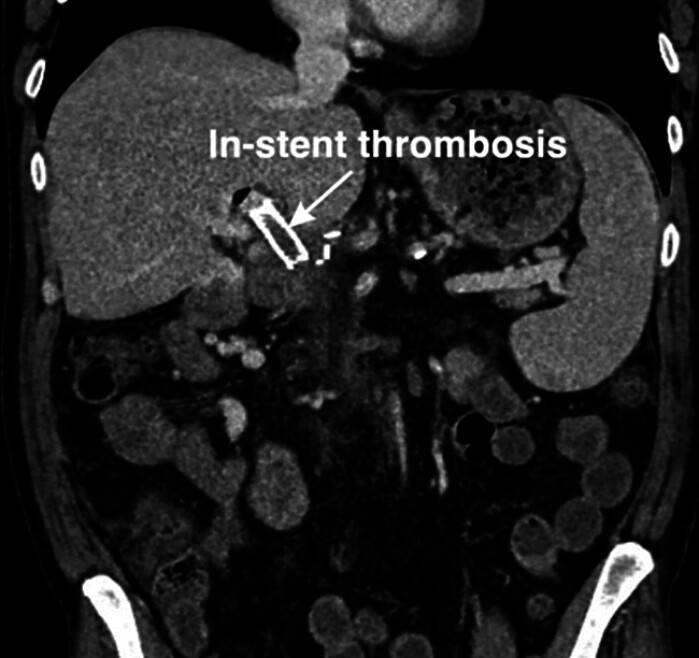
CT imaging confirming In-stent thrombosis.
